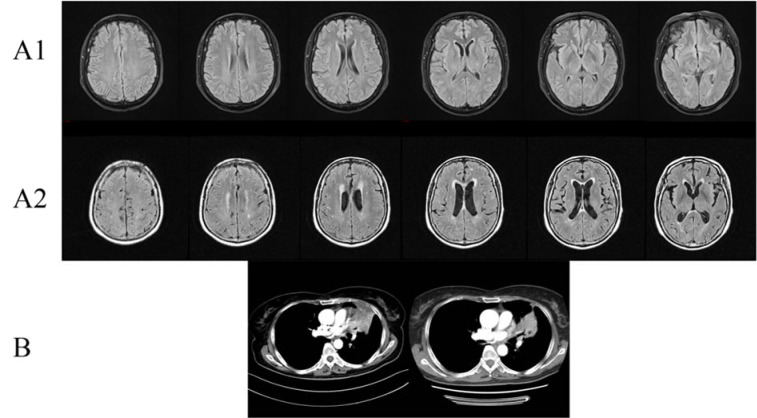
Clinical images of Case 2. (A1) Brain MRI with contrast showed suspicious reinforcement in the epencephalon and some ischemic areas in the frontal, parietal, and occipital lobes before EGFR-TKI therapy; (A2) Brain MRI showed multiple ischemic foci and lacunar infarction, encephalatrophy, and demyelination in white matter after EGFR-TKI therapy; (B) Chest CT with contrast of patient 2 showed partial response in chest lesions after EGFR-TKI treatment.
